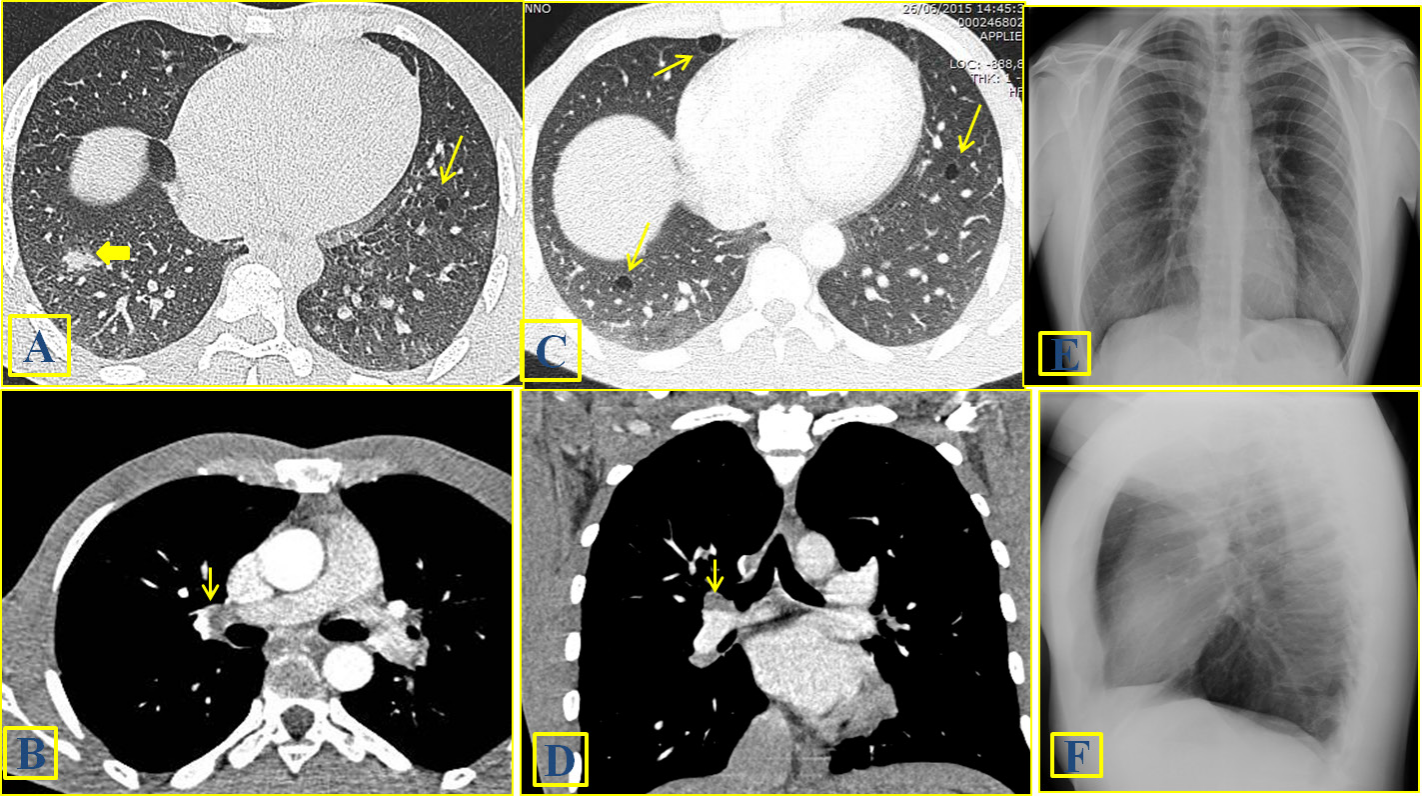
A 29-year-old male suffering from schistosomiasis and abdominal pain. Multiple tiny cystic areas (arrows) can be identified in the lower lobes (5A and 5C). A nodular area (thick arrow) can be recognized on the right side (5A). Two contiguous right hilar adenopathy (arrows) can be identified in the axial (5B) and coronal contrast-enhanced reformatted images (5D). The chest radiographs (5E, 5F) showed no abnormal findings.
